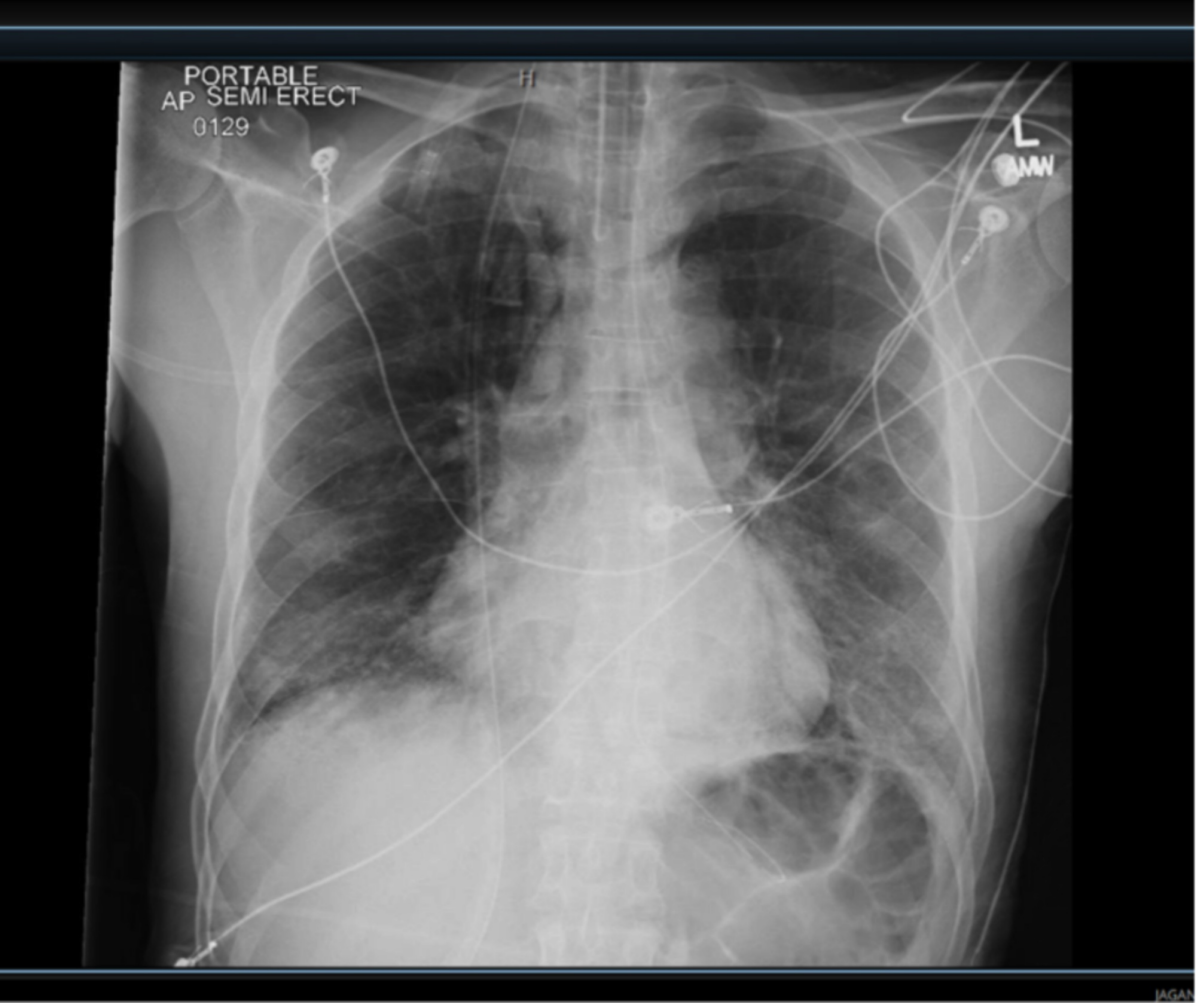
Chest X-ray (CXR) one day post admission demonstrating pneumomediastinum.
